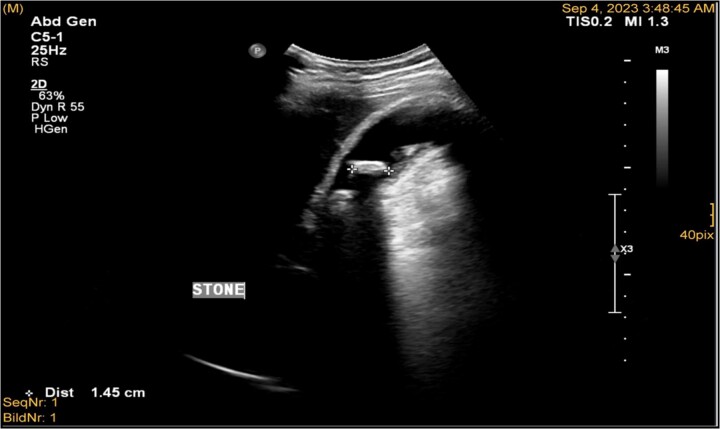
Ultrasonography demonstrated a distended gallbladder, thickened gallbladder wall measuring 6 mm, with multiple gallbladder stones, the largest one measuring approximately 1.4 cm.
